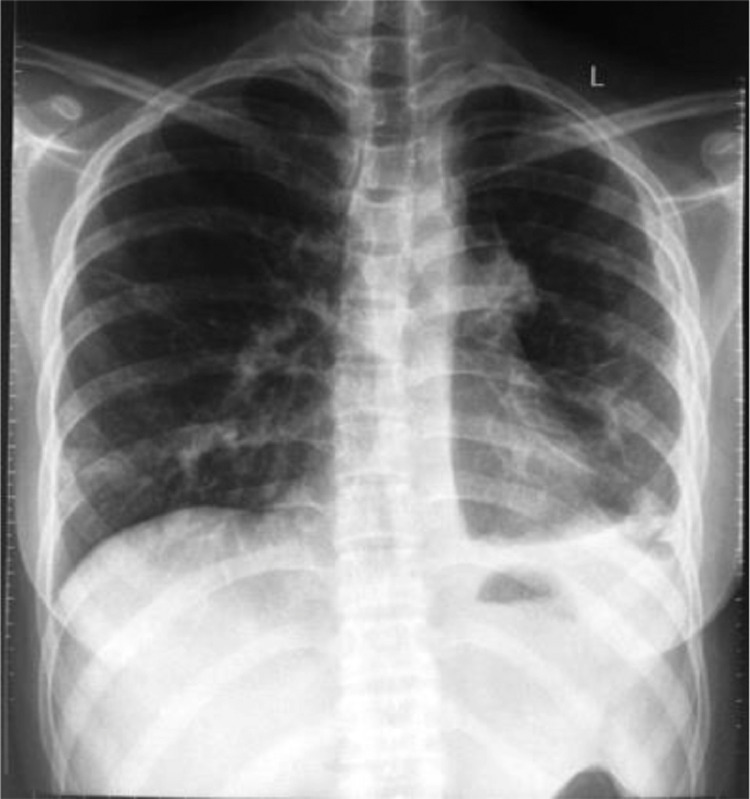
Chest radiograph showing minimal left-sided pleural effusion (star)
